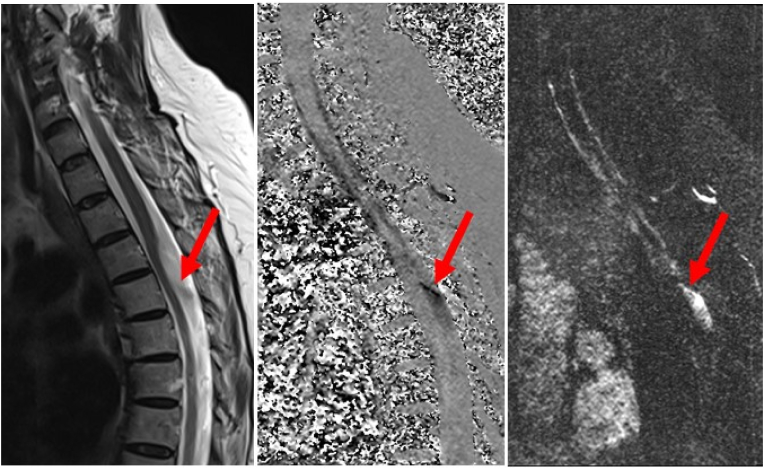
Using cardiac-gated phase-contrast cine-mode MRI, CSF pulsations were found to be interrupted during systole and diastole below the dorsal indentation of the spinal cord, thereby delineating the obstruction of CSF flow caused by the AW.
